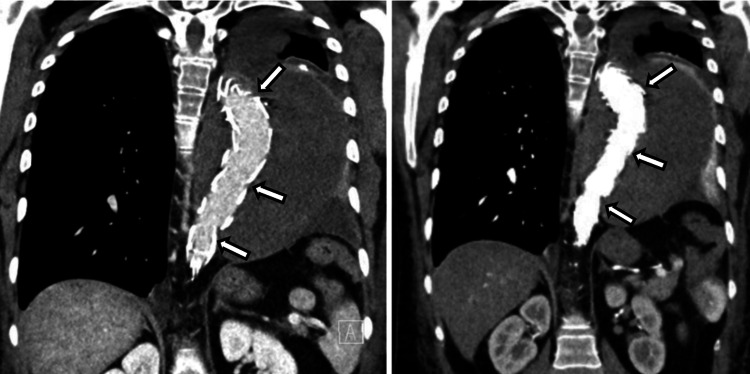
Post-TEVAR CT angiography showing sealed stent graft and no endoleak TEVAR: thoracic endovascular aortic repair; CT: computed tomography
